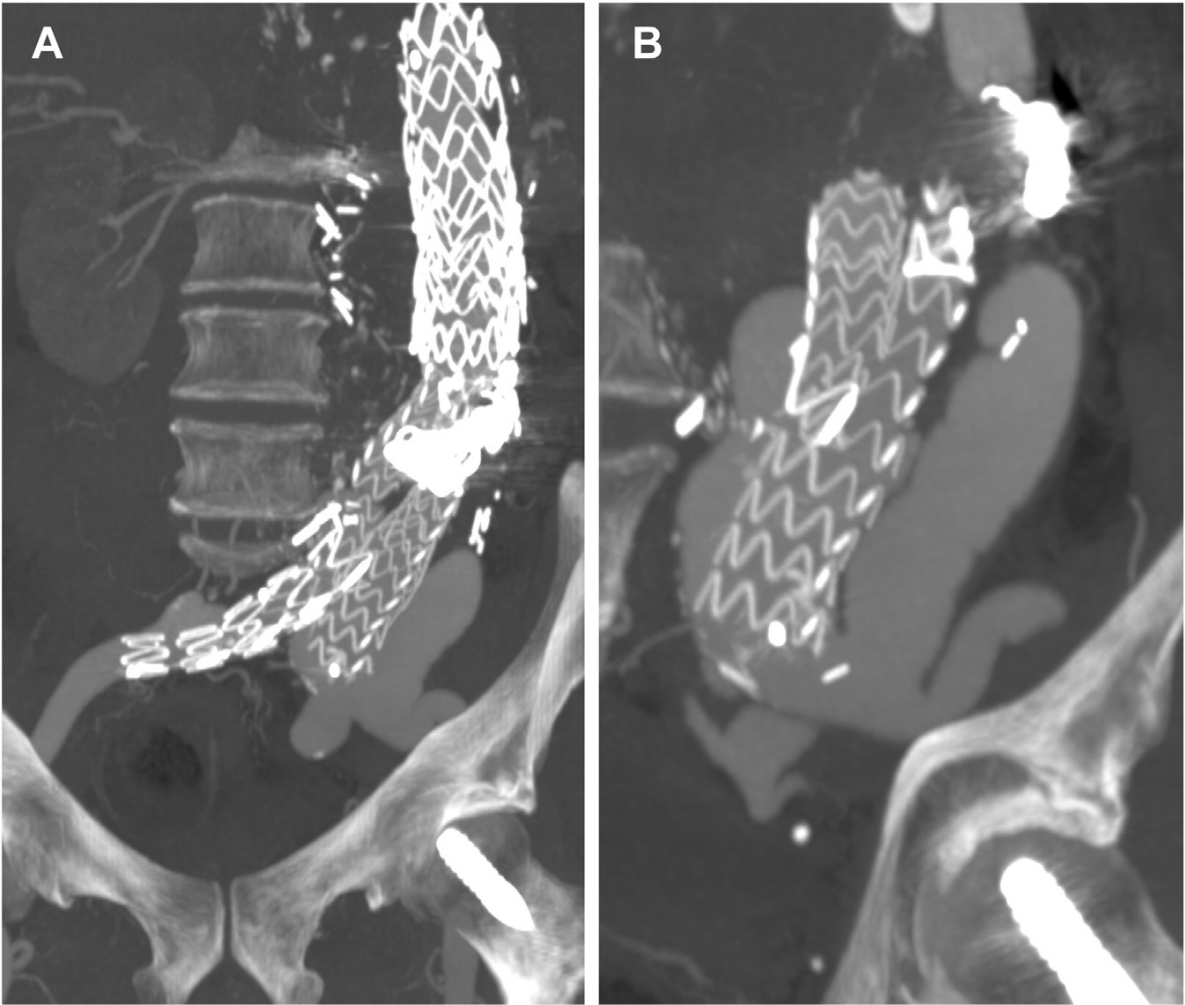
Computed tomography reconstructions evidencing type Ib endoleak through both common iliac arteries. A, Anteroposterior projection. B, Lateral projection showing the relationship with visceral debranching.
